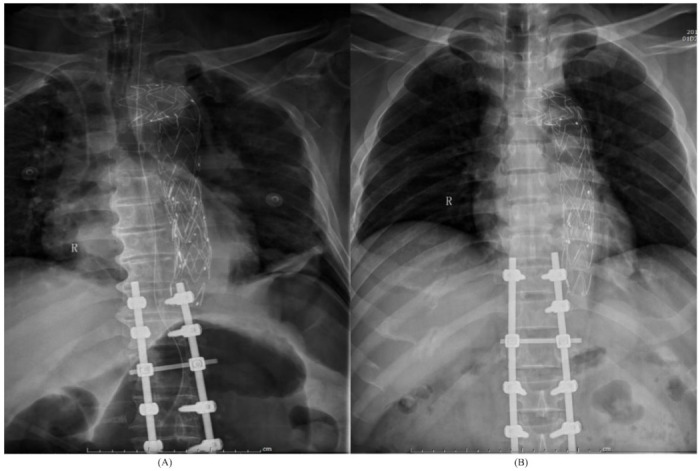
(A) A 48-year-old male was injured in a fall, and suffered from multiple injuries, including multiple rib fractures, hemopneumothorax, T11-T12 burst fractures with paraplegia (ASIA A), grade III aortic injury, and with a history of hypertension and hyperlipidemia for 4 years. Post-operative X-rays showing aortic stent-graft in place with posterior instrumentation of the spine. (B) A 62-year-old male who was involved in a crush injury and suffered multiple injuries, including multiple rib fractures, pneumothorax, T11-T12 burst fractures with paraplegia (ASIA A), Grade III aortic injury, and with a history of hypertension, hyperlipidemia and atherosclerosis. Post-operative X-rays showing aortic stent-graft in place with posterior instrumentation of the spine.
